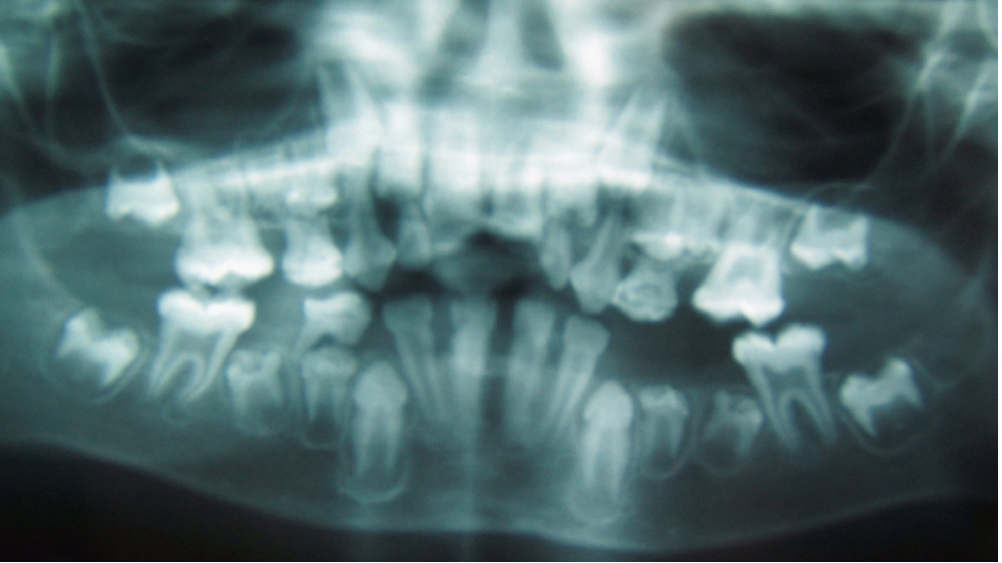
Patient 2: Panoramic radiograph at 7.5 years of age. The radiograph reveals enlarged pulp chambers and abnormalities of the shape of the crowns.
